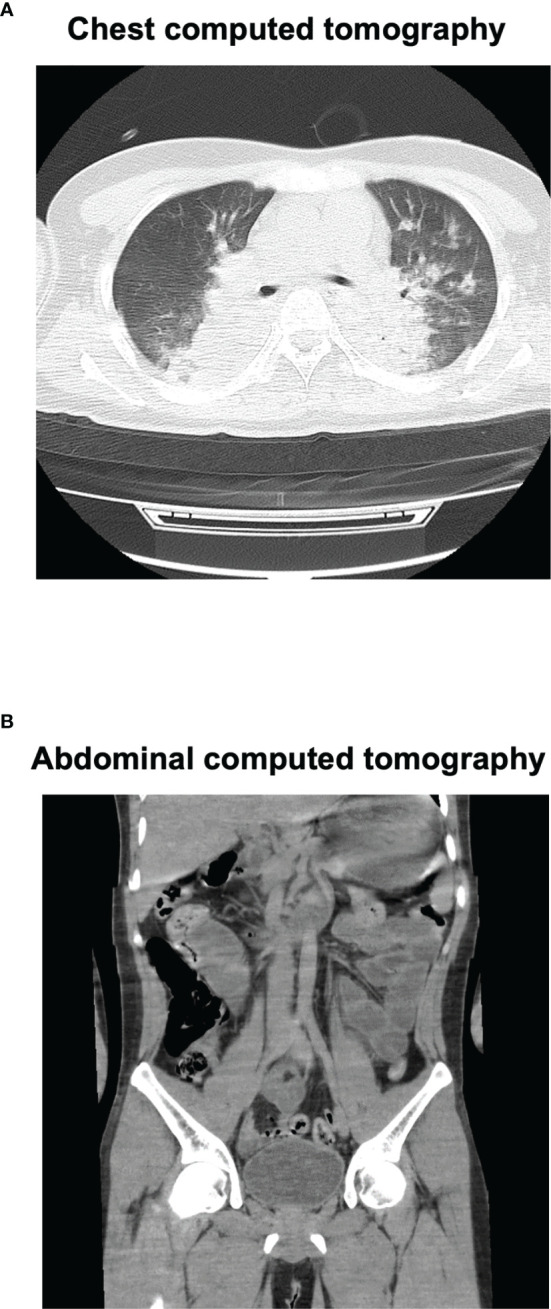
Chest and abdominal computed tomography (CT) on admission. Chest CT (A) revealed marked pleural effusion and pulmonary edema. Abdominal CT (B) revealed marked intestinal edema.
